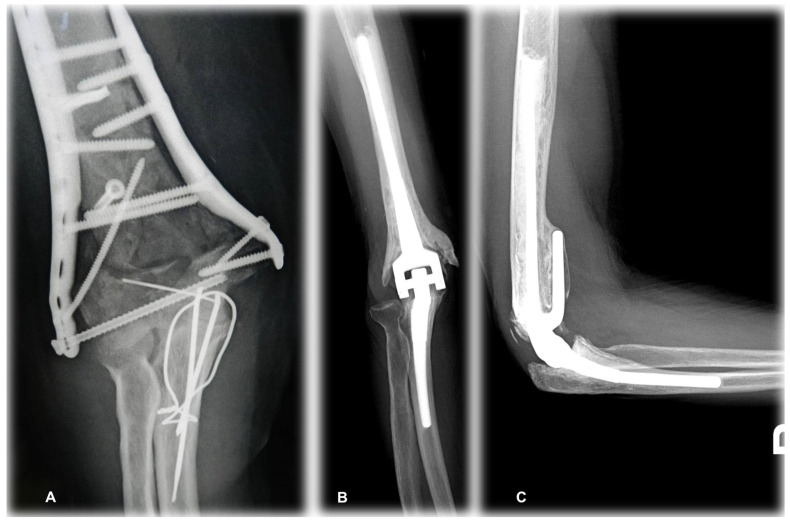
Preoperative (A) and Postoperative (B,C) X-rays (Case 4).
